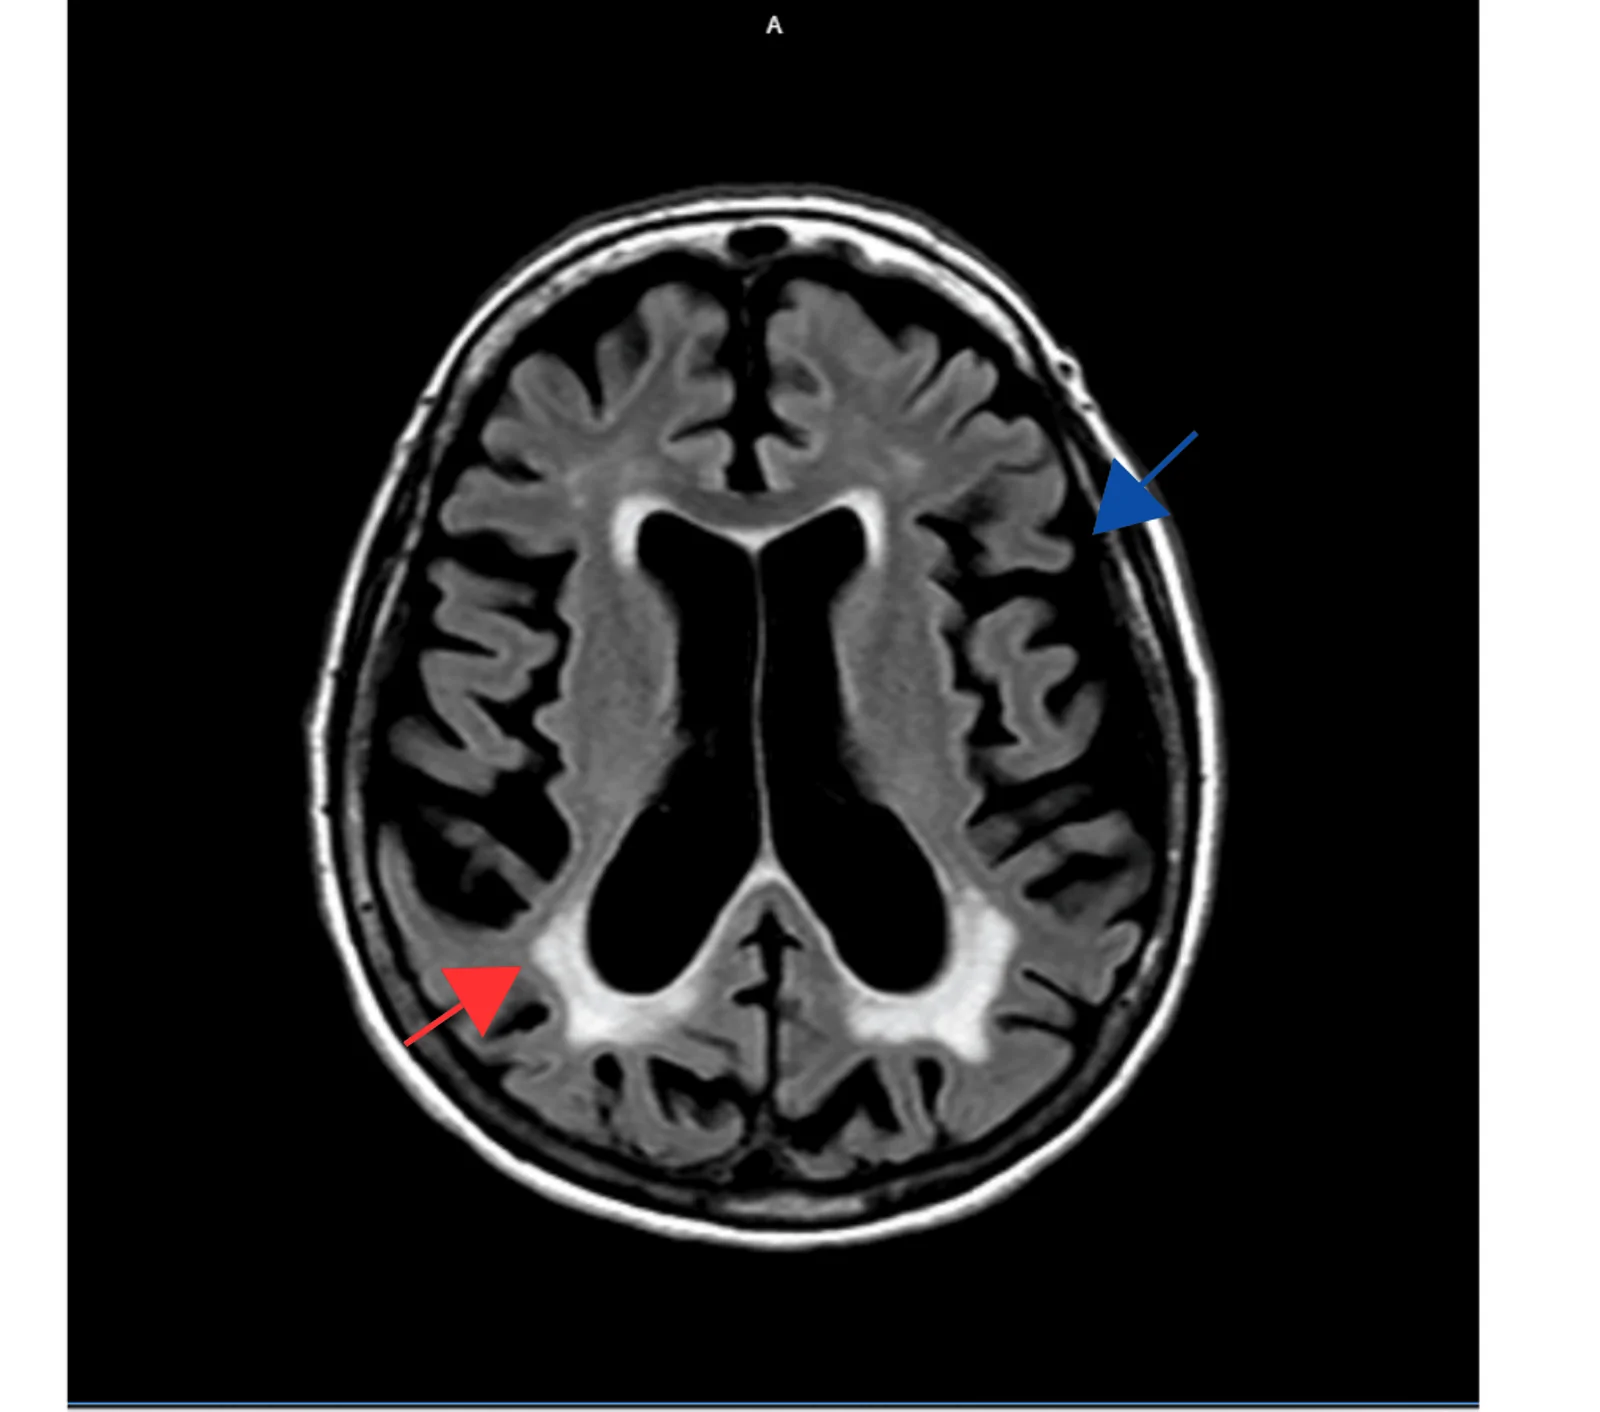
MRI brain: axial T2-FLAIR (fluid-attenuated inversion recovery) Axial MRI demonstrates global cerebral volume loss (blue arrow) and nonspecific periventricular and subcortical white matter hyperintensities (red arrow), consistent with chronic white matter changes.
